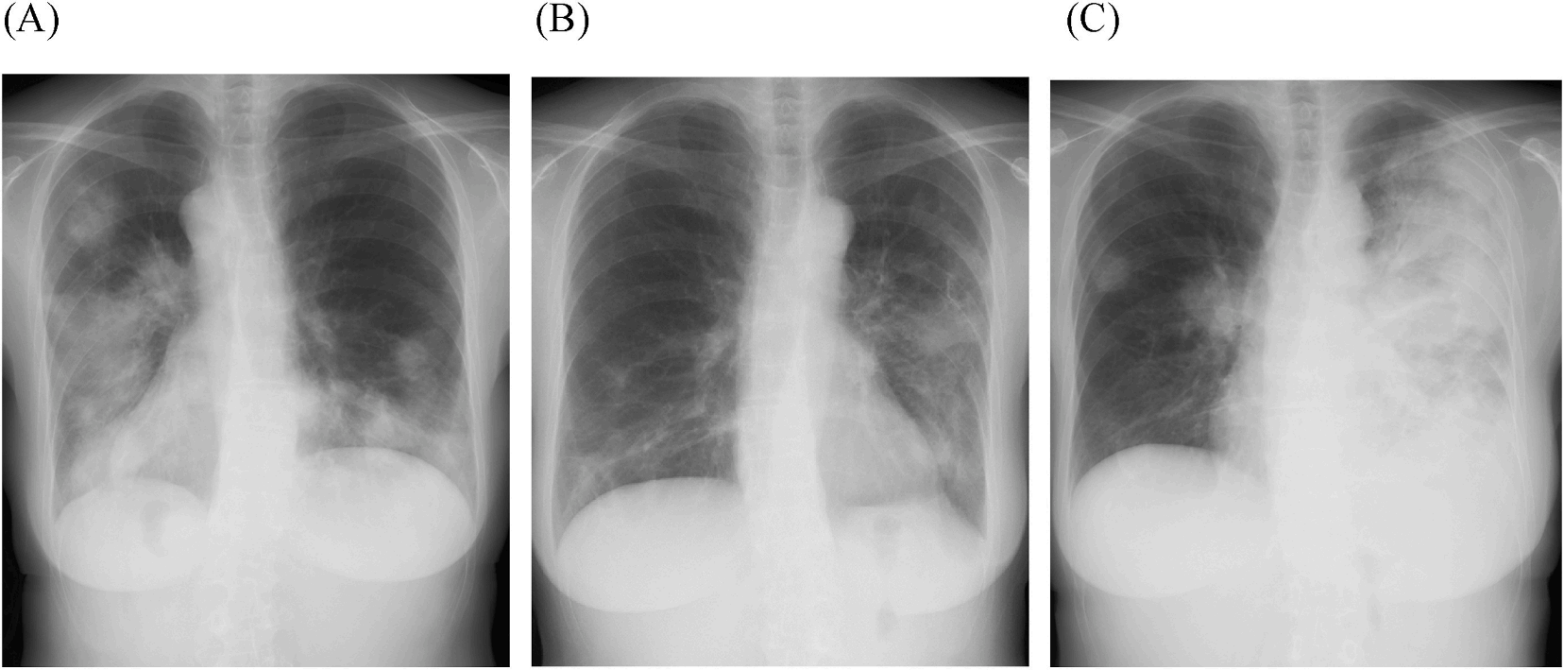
Chest X-rays of the patient. (A) Image at first visit to our hospital showed bilateral multiple tumorous and nodular shadows. (B) Image obtained 3 months after first visit showed improvement of opacities in the right lower lung field, and nodular shadows were partly enlarged in the left lung. (C) Image at 7 months after first visit showed extensive consolidation and enlargement of tumorous shadow in the left lung field. Nodular shadows were also present in the right hilum and the middle right lung field.
